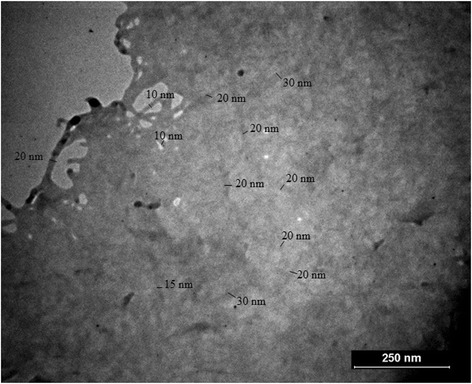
TEM images of nanocellulose prepared by hydrolysis with H_2_SO_4_ concentration 43% at 40 °C and ultrasound treatment for 30 min
